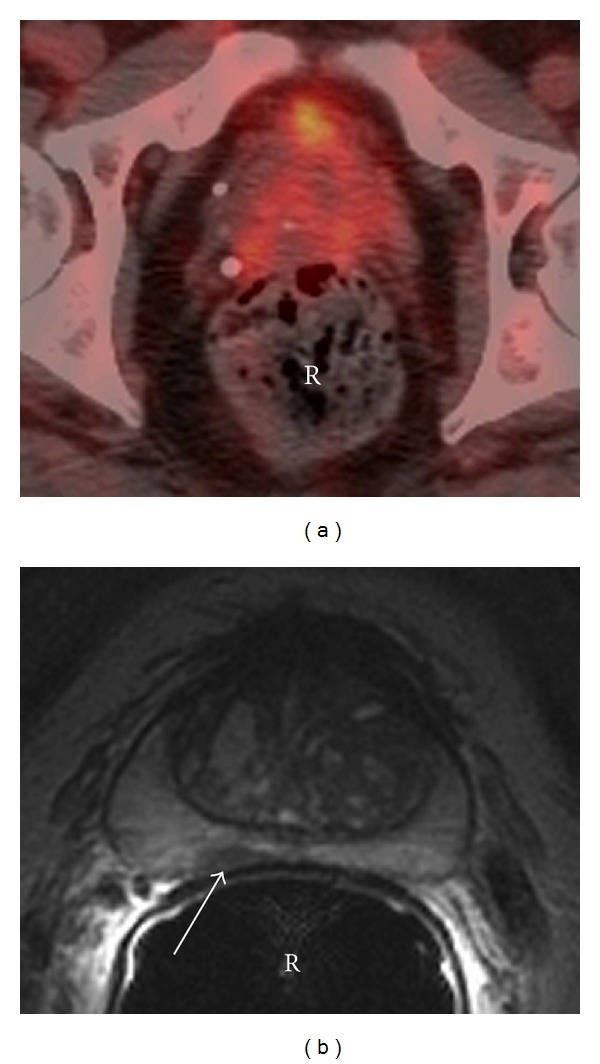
60-year-old male with history of Hodgkin lymphoma. (a) Axial fused PET/CT image shows diffuse uptake in the prostate. (b) Axial T2 weighted sequence through the prostate with an endorectal coil (EC) shows focal decreased signal in the peripheral zone posteriorly (arrow) anterior to the rectum (R). Biopsy of the prostate gland demonstrated prostate adenocarcinoma in the right and left base and the apex with a Gleason score ranging from 6 to 8.
